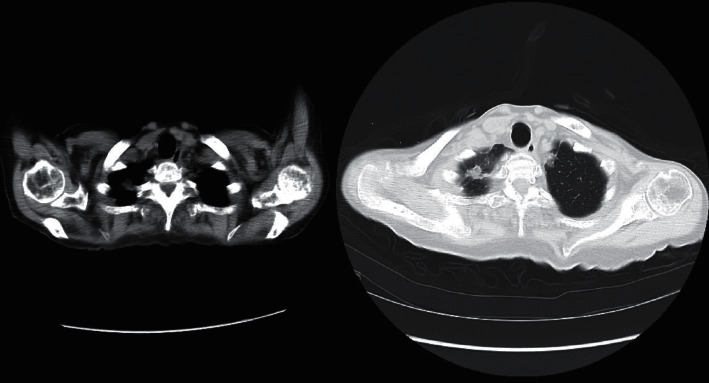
Chest CT showing thickening of the pleura and a nodule at the apex of the left lobe.
